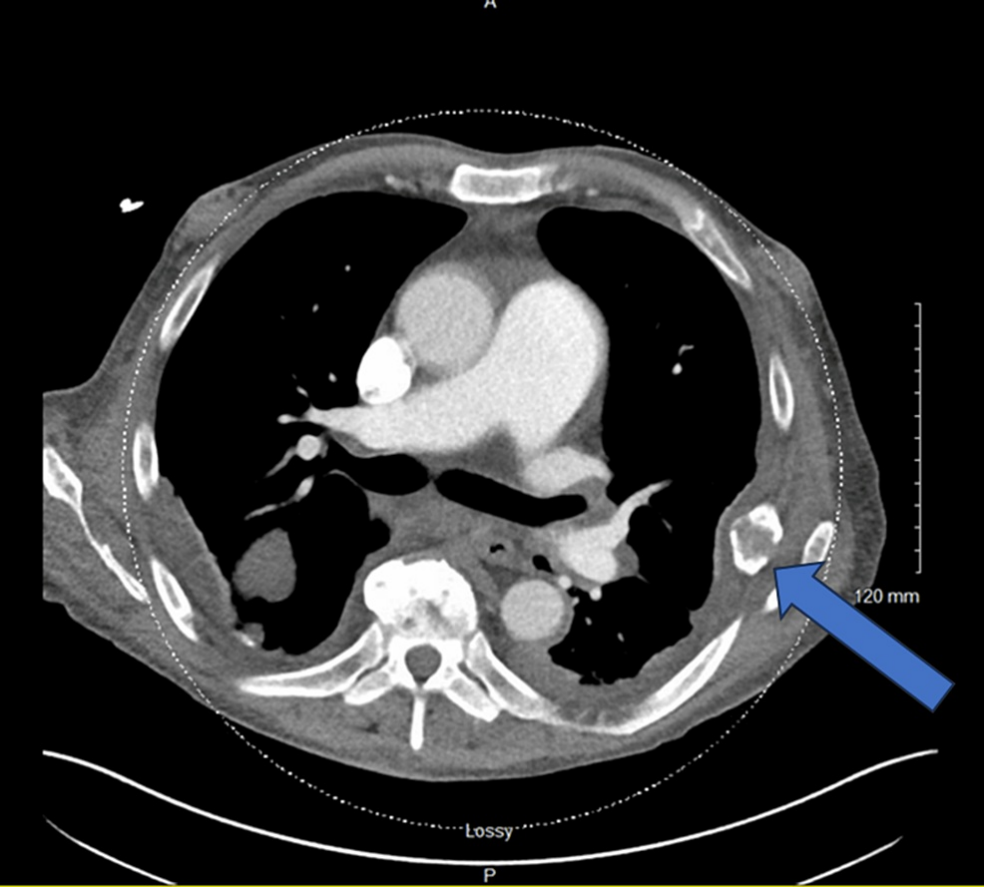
CT chest showing left sixth rib lytic lesion.
